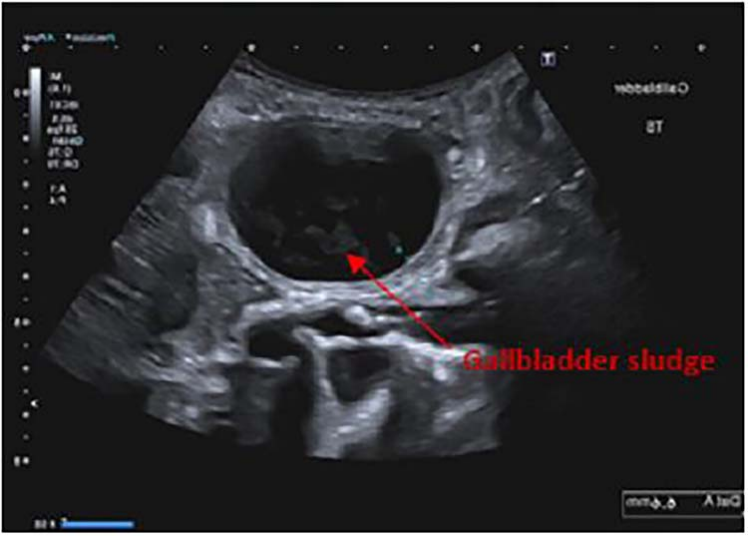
Ultrasound abdomen showing gallbladder sludge.
